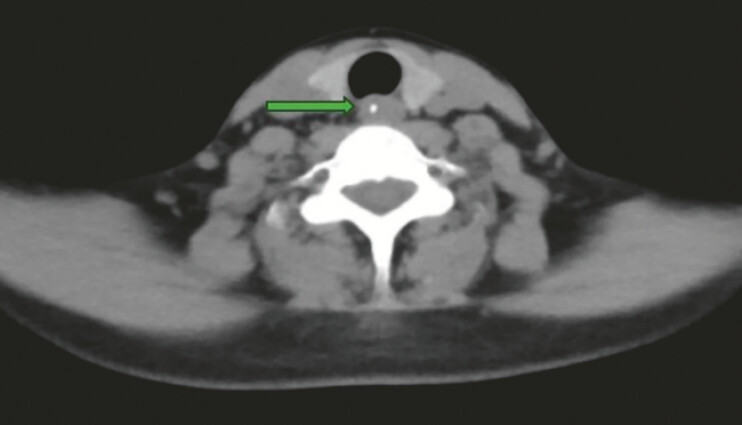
Cervical computed tomography showing a linear hyperdense lesion (~2.3 cm) in the upper esophagus at the C6–C7 level, with esophageal wall thickening and luminal narrowing, suggestive of an esophageal foreign body.
